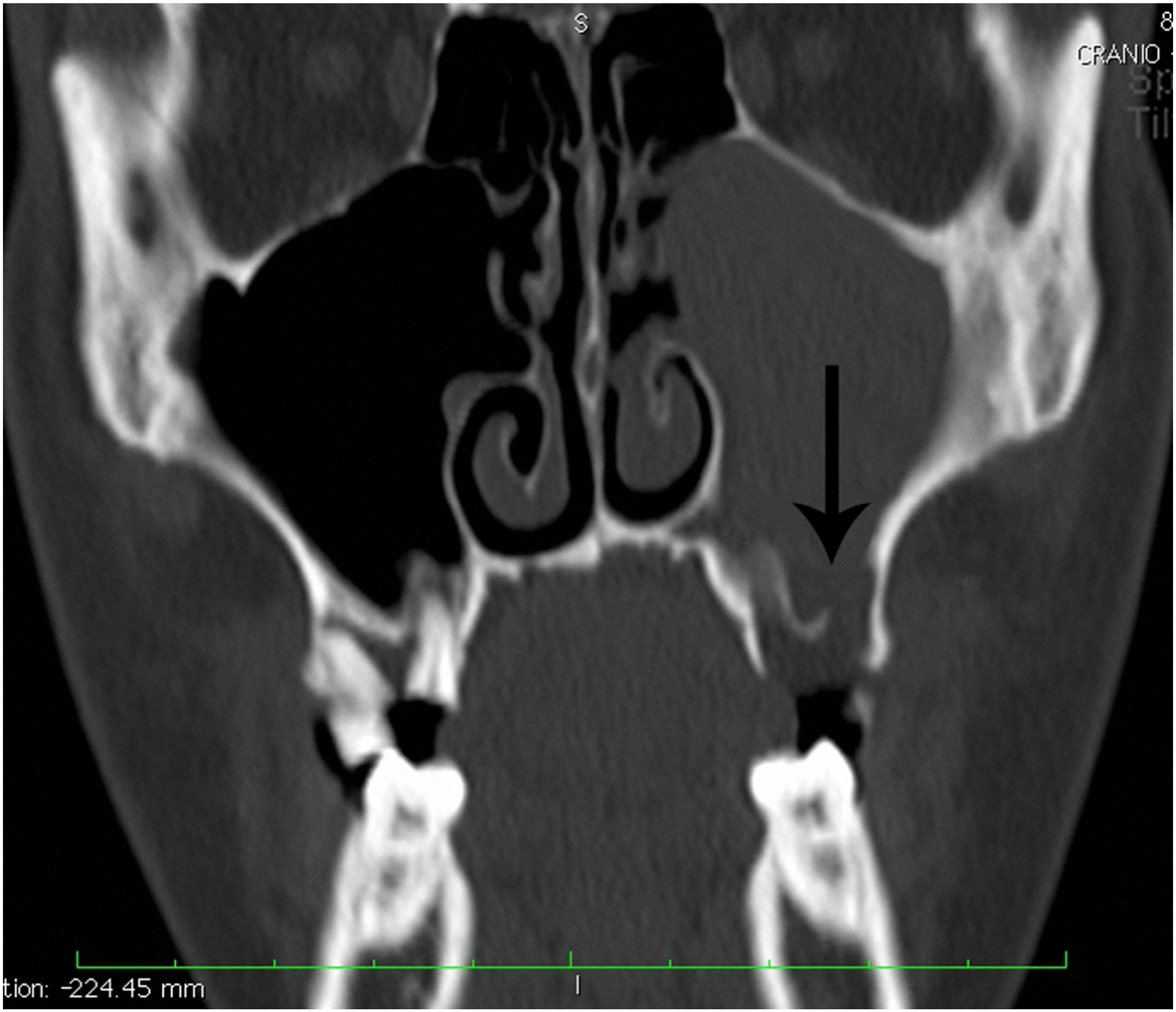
Coronal computed tomography scan showing the interruption of the sinus floor (black arrow) corresponding to alveolar process secondary to tooth extraction.
